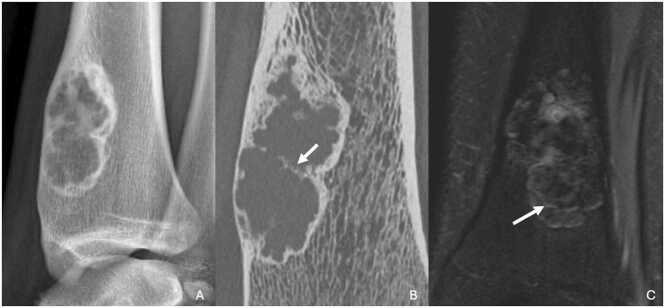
Cortical defect of the left tibia in a 19-year-old young man incidentally discovered in extension study of SAMS bacteremia. A. Profile radiography of the left tibia showing oval radiolucent lesion with sclerotic margins centered on the cortex of the tibial distal metaphysis. B, CT image in the sagittal plane highlighting this osteolytic lesion with sclerotic margins, with some internal septa (white arrow). C. T2-weighted fat-suppressed MR image in the coronal plane showing a heterogenous signal of the lesion, mainly in T2 hyposignal with internal septa in T2 hypersignal (white arrow).
